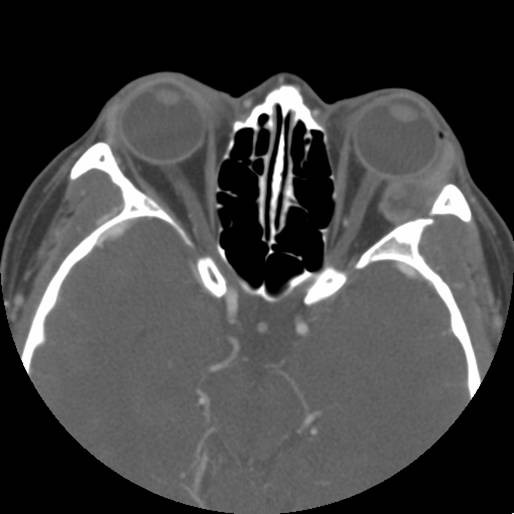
Contrast-enhanced axial orbital computed tomography showing a well-circumscirbed mass at lateral aspect of the left orbit without bone involvement.
